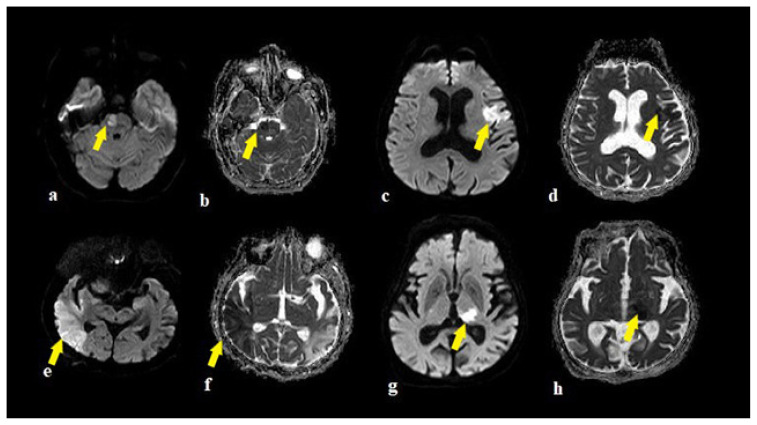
The examples of infarctions in a 64 years old female in pons (a,b), in a 68 years old man in Broca region (c,d), in 43 years old man right MCA territory (e,f), in a 32 years old man in left talamus (g,h) on DWI and ADC images (arrows).
